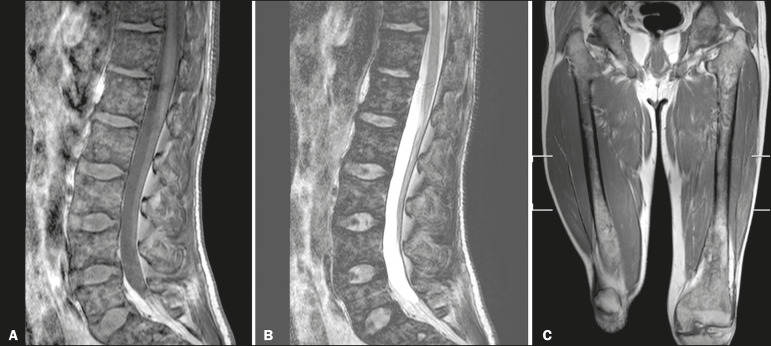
MRI of a 40-year-old man with GD who, despite having extensive bone involvement, did not show poor quality of life, as measured with the SF-36. Sagittal T1-weighted (A) and T2-weighted (B) images of the lumbar spine, showing lower signal intensity in bone marrow than in the disc and presacral fat. The signal intensity score was 5, and the extent score was 1 because the fat surrounding the basivertebral veins was not suppressed, resulting in an L-BMB score of 6. C: Coronal T1-weighted image of both femurs, which were scored 5 for signal intensity and 3 for the extent of involvement, infiltration of epiphyses being detected, resulting in an F-BMB score of 8.
